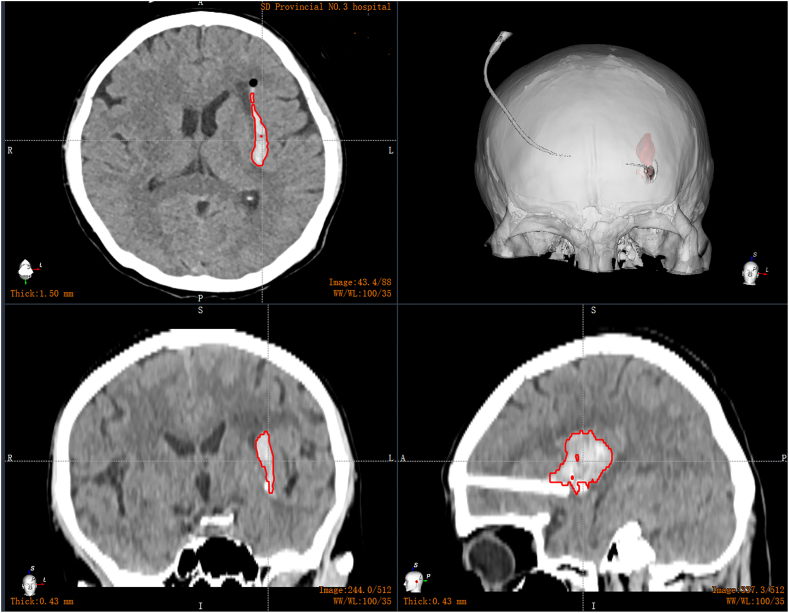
The postoperative CT of case 1.
